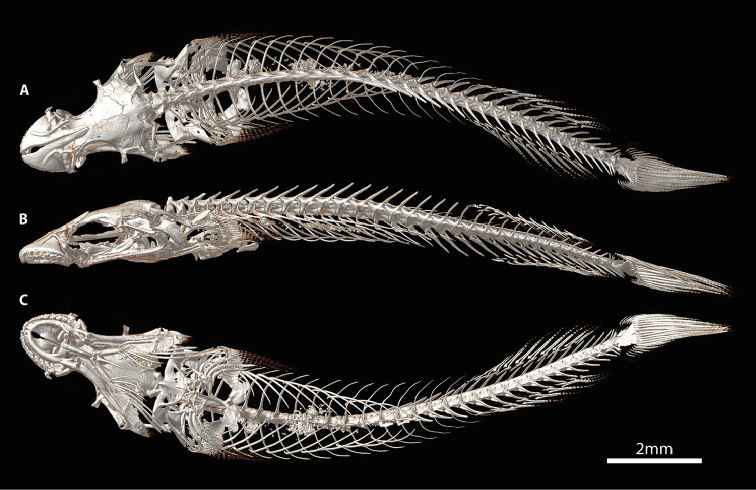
CT scanned skeleton of *Flexorincus*, AIM MA655316, paratype, 23.0 mm SL. A Dorsal view. B Lateral view (left side) C Ventral view.
